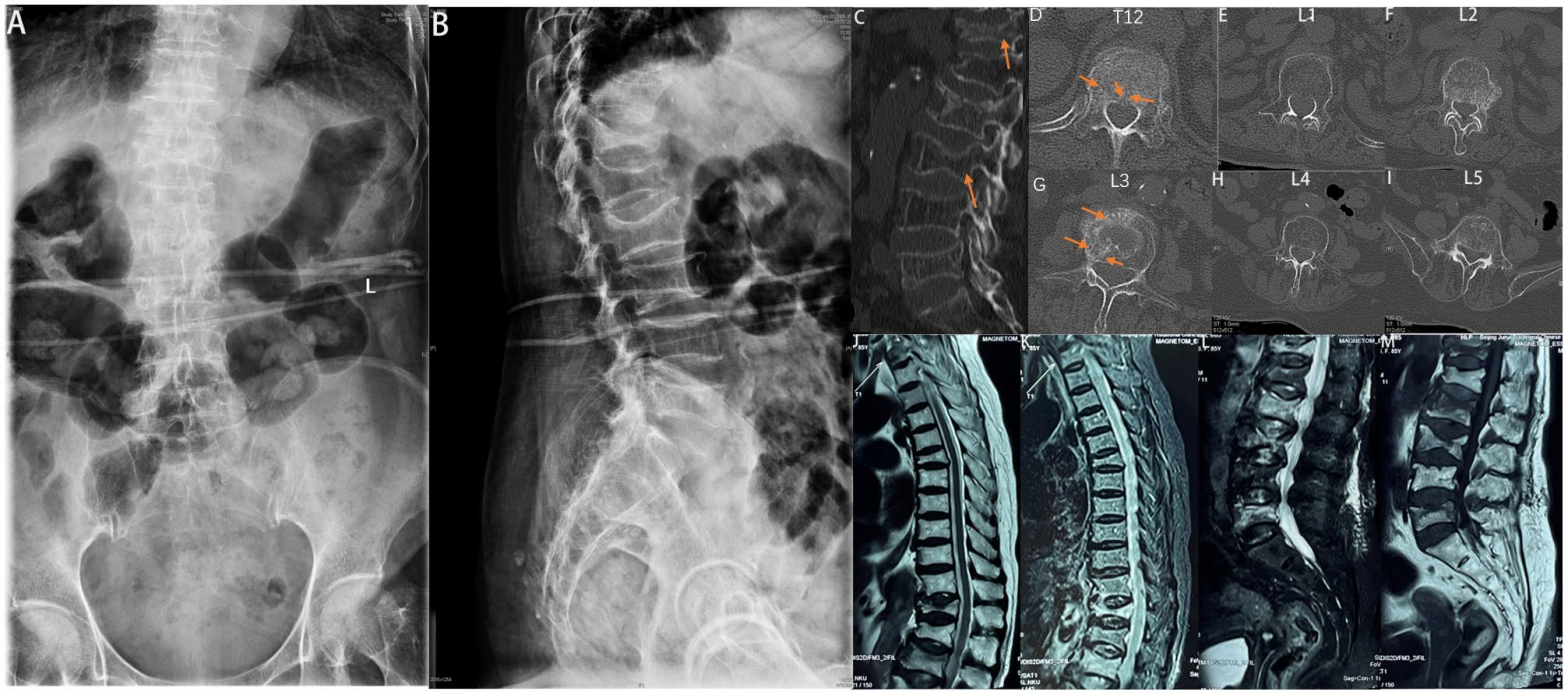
Preoperative radiograph of the spine. (A) Anteroposterior and (B) lateral radiographs showing T12 and L5 vertebral compression fractures and L2 and L3 vertebral burst fractures. Preoperative CT of the spine (C−I). T12 and L5 vertebral compression fractures, and L2 and L3 vertebral burst fractures. Preoperative MRI of the spine (J−M). T12 and L5 vertebral compression fractures, and L2 and L3 vertebral burst fractures.
